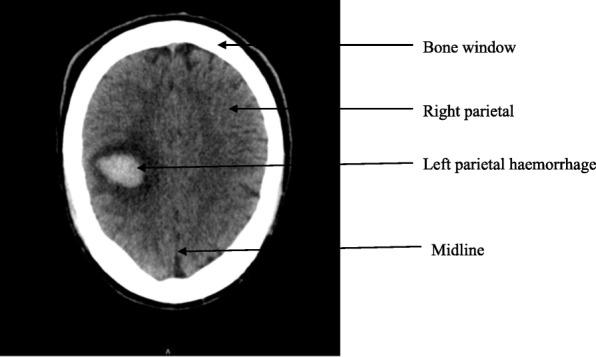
Emergency cerebral CT scan showing spontaneous left parietal haemorrhage
